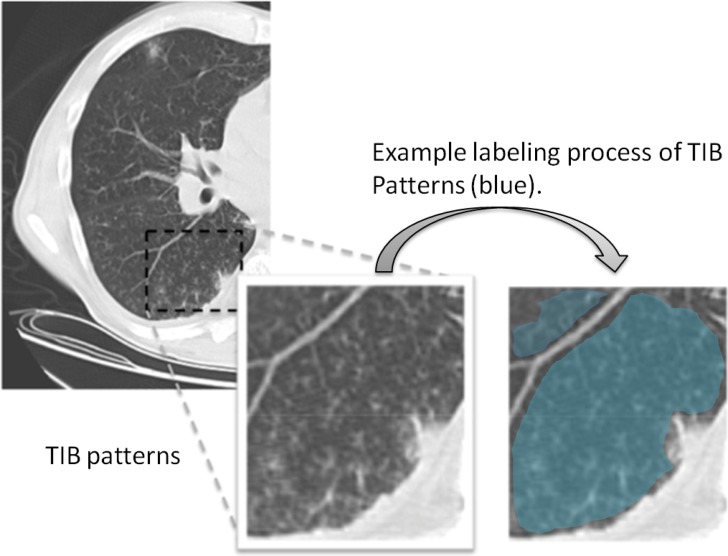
Single-axial CT slice with a significant amount of TIB patterns and an example labeling process of TIB patterns (blue) on the right lung are shown.
